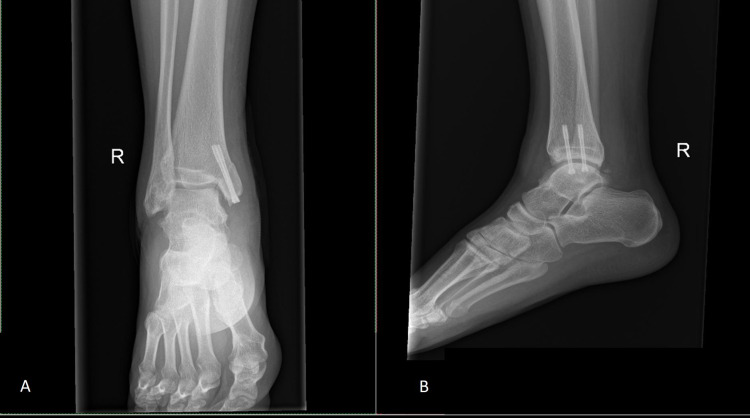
Immediate post-surgery plain radiograph of AP (A) and lateral (B) view of the right ankle show screw fixation of the medial malleolus. AP: Anteroposterior
